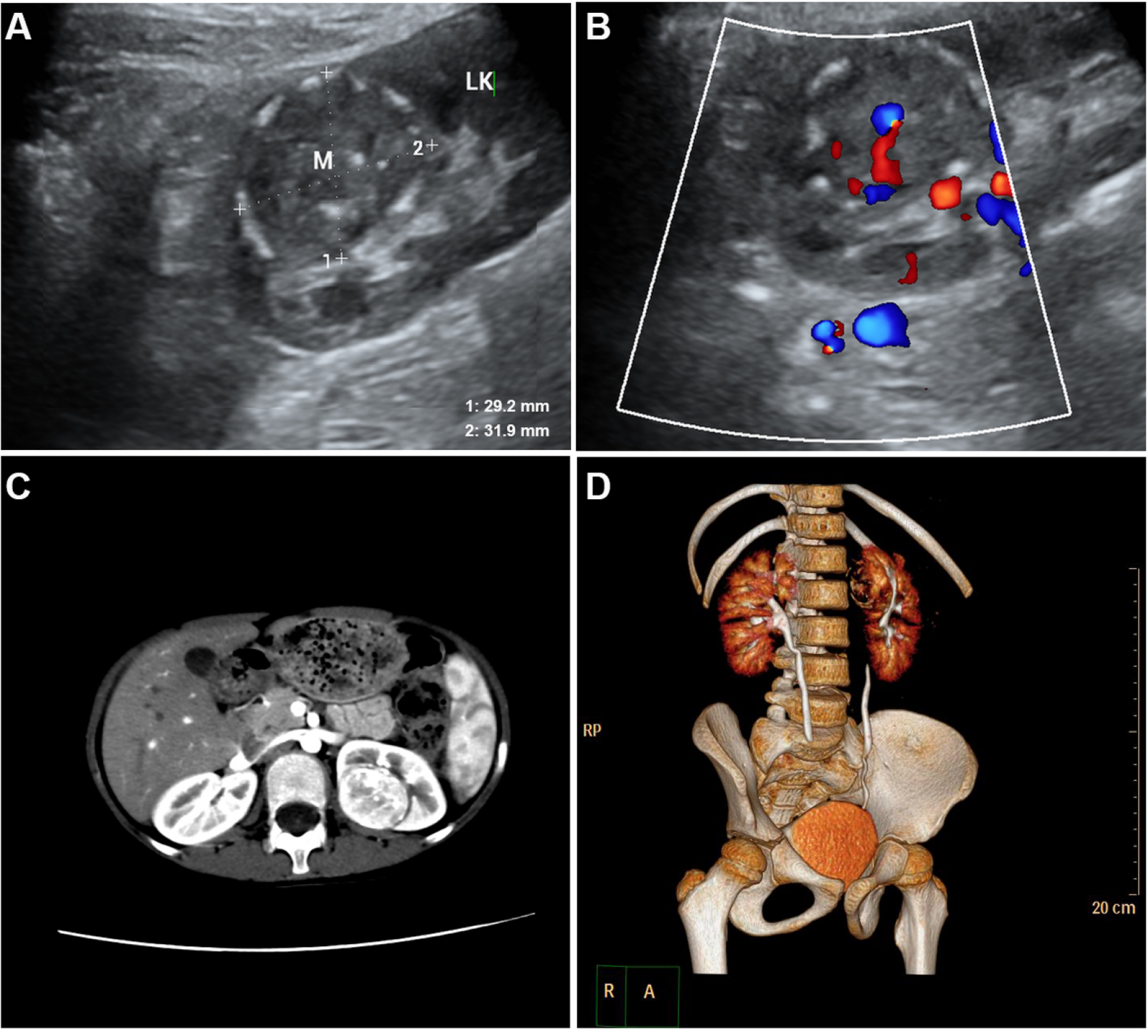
Two-dimensional ultrasound showed a solid hypoechoic mass at the upper level of the left kidney with clear boundary and annular calcification around (A). CDFI showed abundant blood flow signals (B). Contrast enhanced CT showed heterogeneous enhancement mass of left renal parenchyma (C). 3D imaging showed that the mass was located on the superior dorsal side of the left kidney, and some renal calyces were compressed and narrowed (D)
